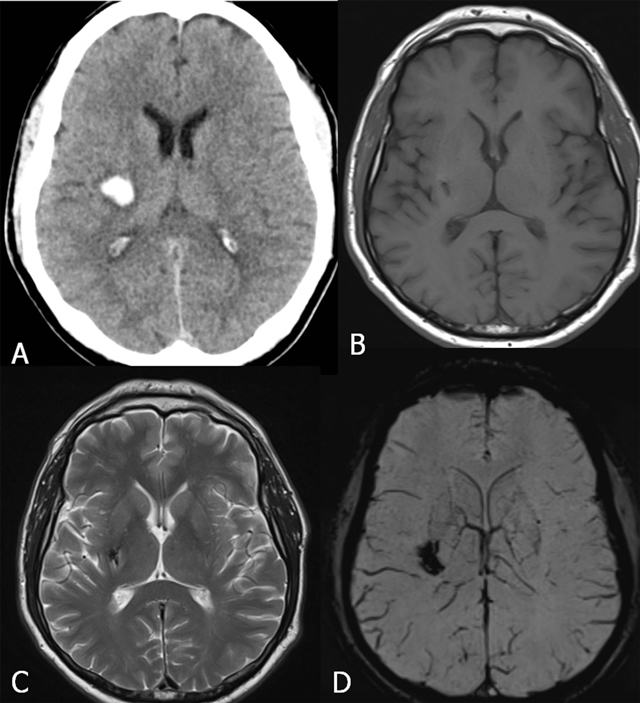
Hypertensive central hemorrhage in patient with severe hypertension. CT (acute fase) (A) and follow-up MRI with SE T1-WI (B), TSE T2-WI (c) and SWI (D). Acute cerebral hemorrhage (A). Parenchymal defect (B) and focus of low signal with susceptibility-induced signal loss (C, D) best seen on SWI.
